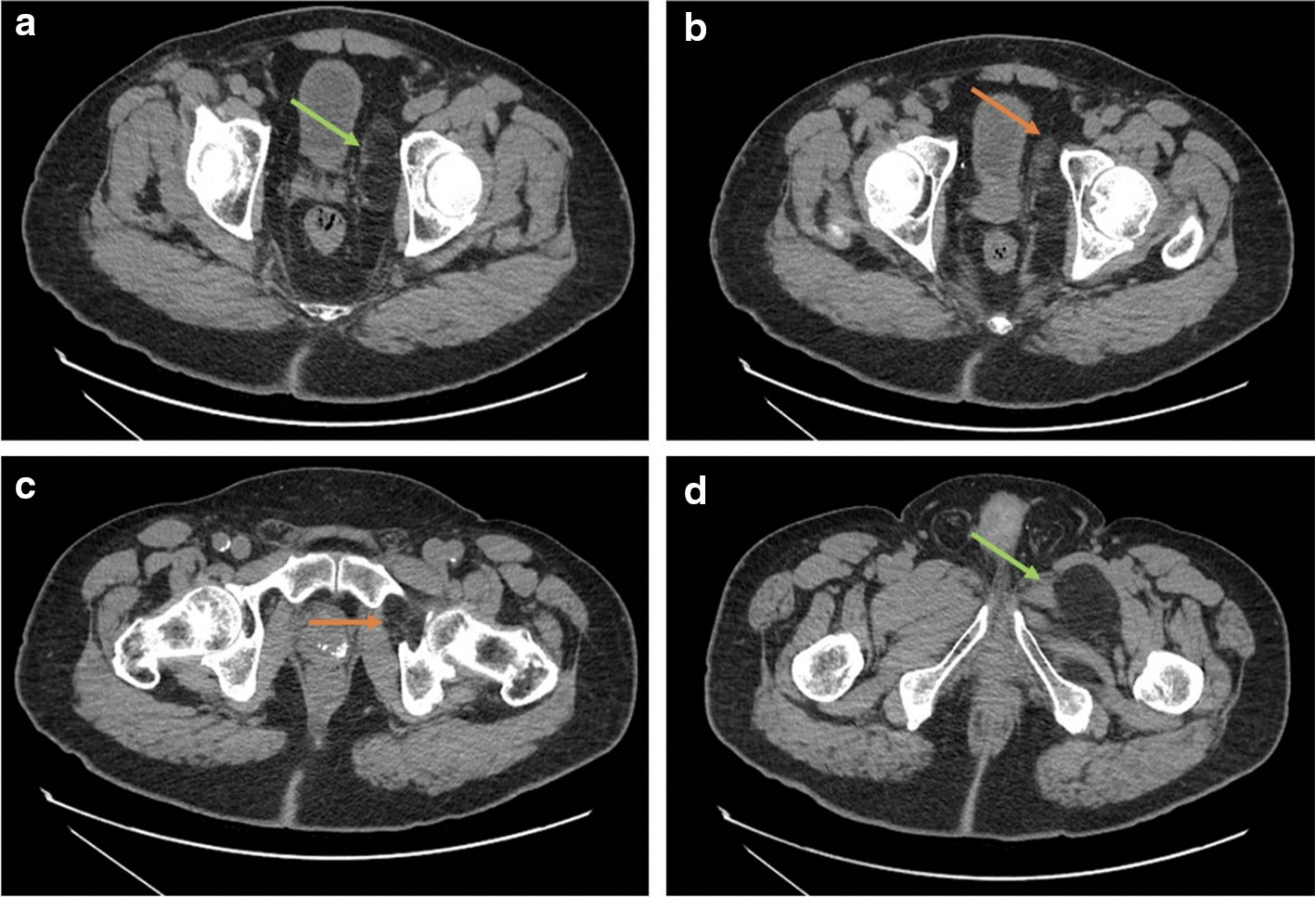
(a – d) CT images with axial views demonstrating the fat density lesion extending from the obturator canal into the left thigh. Green arrows demonstrating normal fat. Orange arrows demonstrating abnormal aggressive features. 2b. A solid soft tissue nodule is seen within the lesion. 2c. Septations areseen within the lesion.
